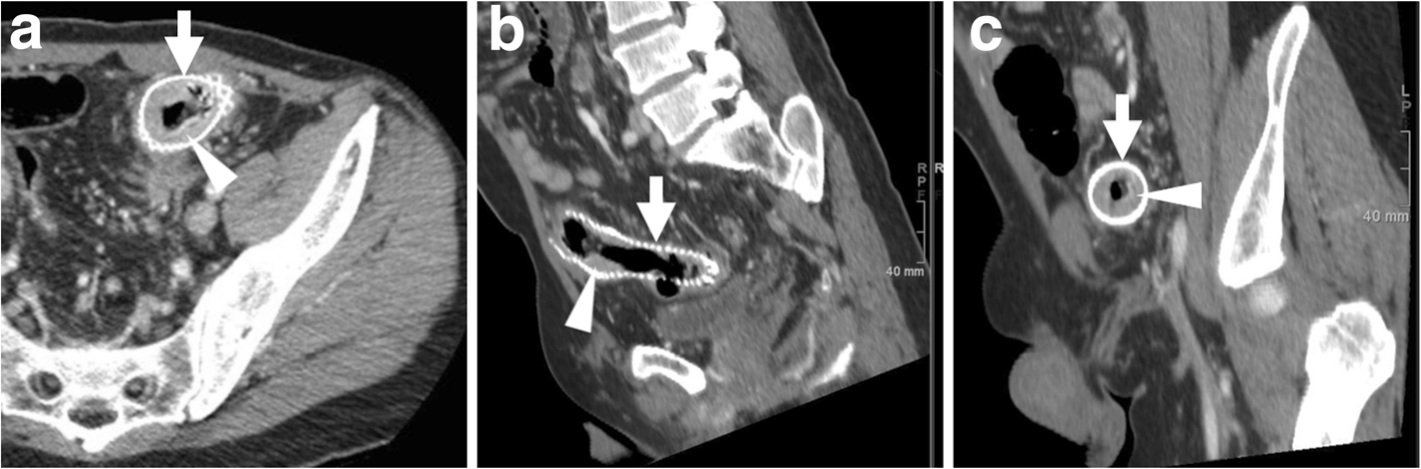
Tumour ingrowth causing stent malfunctioning. In the same patient, compared to initial MRI (Fig. 5), focused multiplanar reconstructions (a-c) of follow-up CT showed enhancing neoplastic tissue (arrowheads) growing within the SEMS (thick arrows), leading to recurrent LBO
